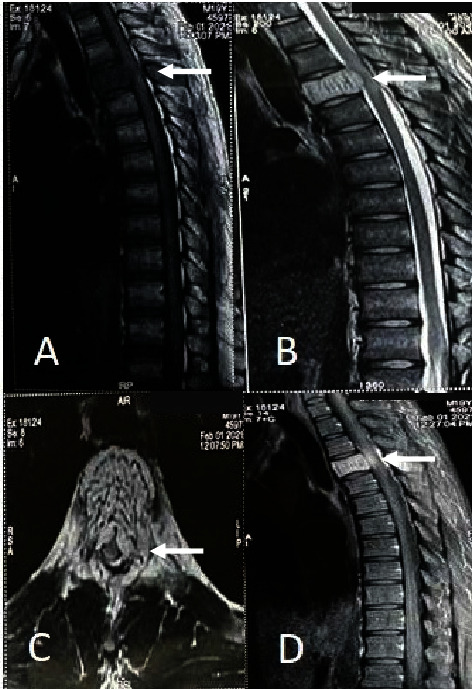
MRI findings. (A) T1 and (B) T2 sagittal images showed hypointense and hyperintense signals in the body and posterior elements, respectively. (C) T2 weighted axial image at T4 vertebrae showing increased signal intensity with cord compression (D) Contrast-enhanced sagittal image showing lesion with soft tissue extension posterior to the body causing almost circumferential compression of the cord.
